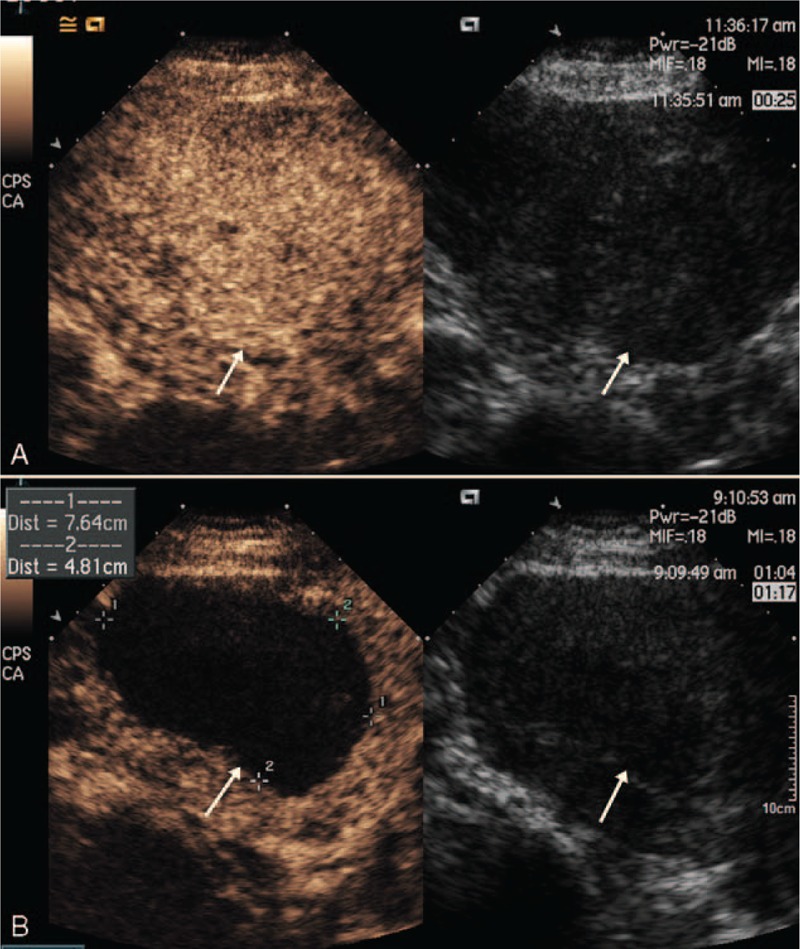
A 45-year-old woman who underwent USgHIFU for symptomatic adenomyosis. (A) CEUS obtained before USgHIFU showed inhomogeneous enhancement (arrows) of the posterior wall of the uterus. (B) Immediate CEUS obtained after treatment showed a nonenhanced region (191 cm^3^) and an NPV ratio of 68.7% in the treated adenomyosis. The margin of the nonenhanced region (arrows) was showed at the ablated region. CEUS = contrast-enhanced ultrasonography, NPV = nonperfused volume, USgHIFU = ultrasound-guided high-intensity focused ultrasound.
